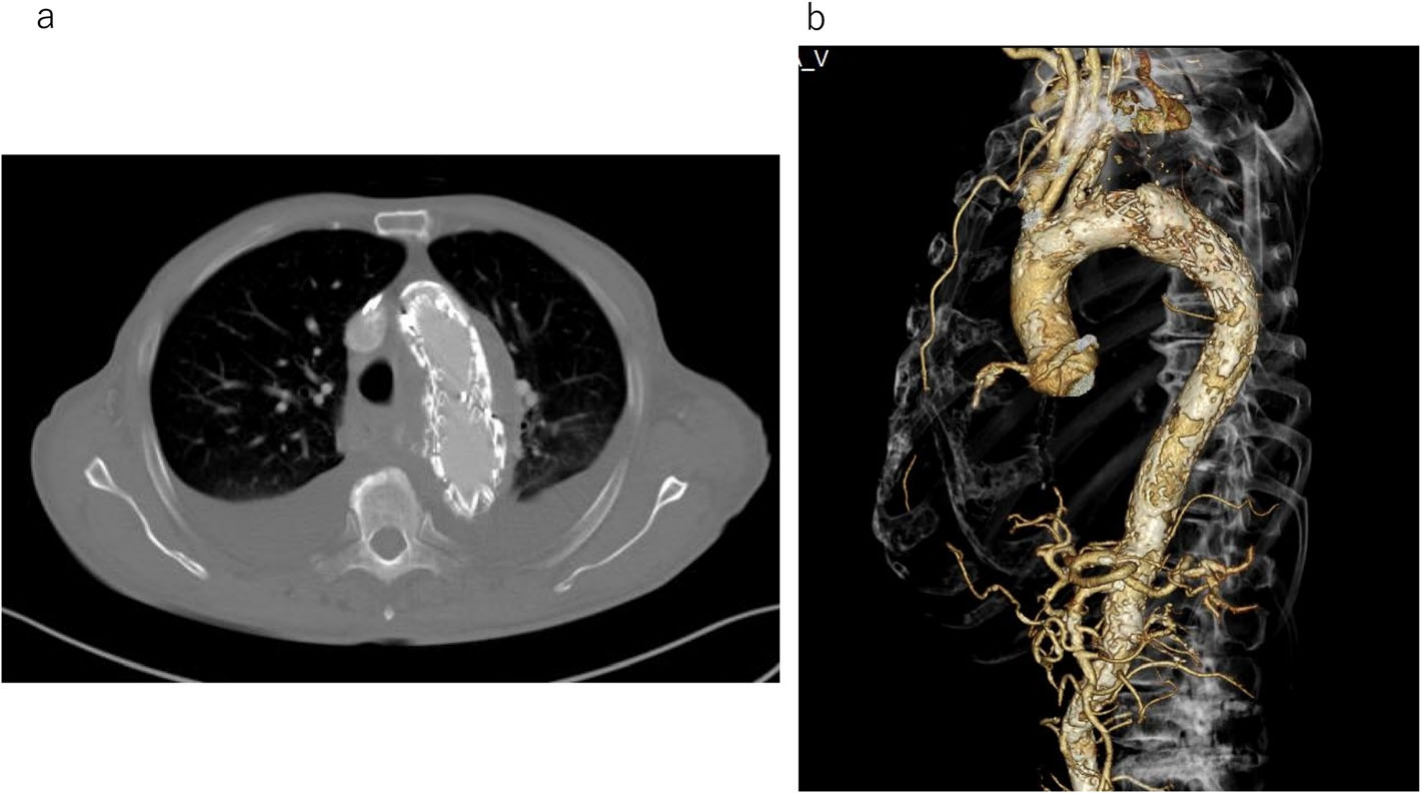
a Postoperative enhanced CT. No leakage is detected, and the aneurysm is shrinking in size. b No retrograde type A aortic dissection is detected
